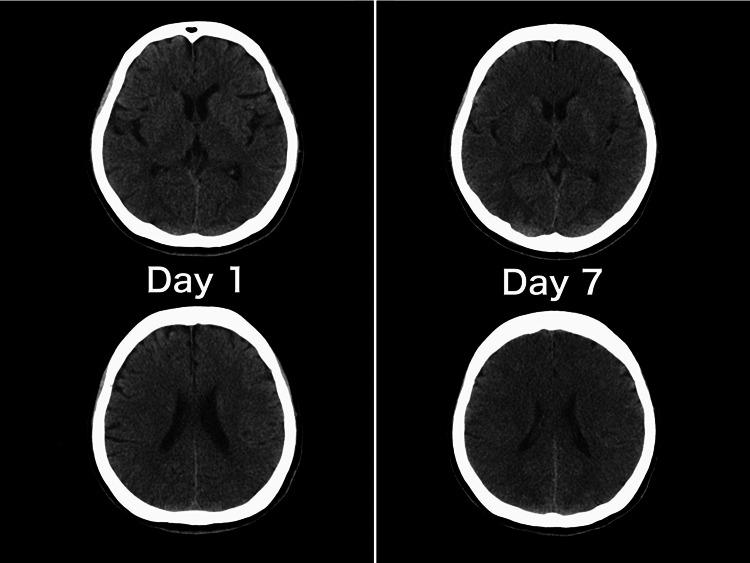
Computed tomography of the brain at day 1 and day 7. Plain head computed tomography on the first day showed no abnormalities. Findings such as narrowing of the cerebral sulci and bilateral ventricles with loss of gray-white differentiation in the temporal and frontal areas and relatively low parenchymal density suggest cerebral edema on day 7.
